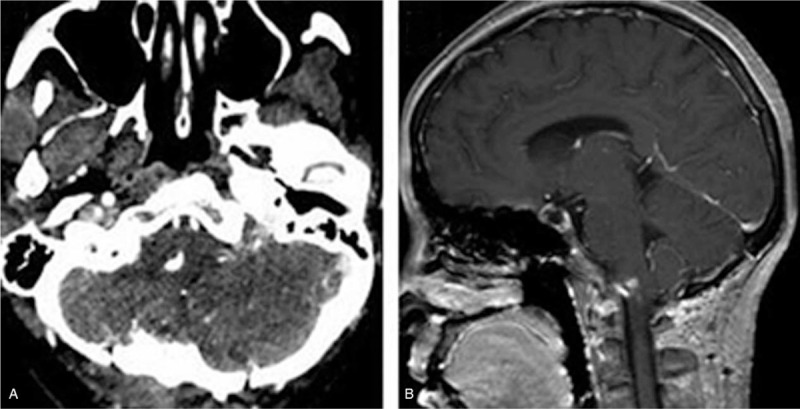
(A)Postoperative CT showing a subtotal resection of the lesion. (B) Follow-up brain MRI showing that residual lesion remained stable at 28 months after surgery.
